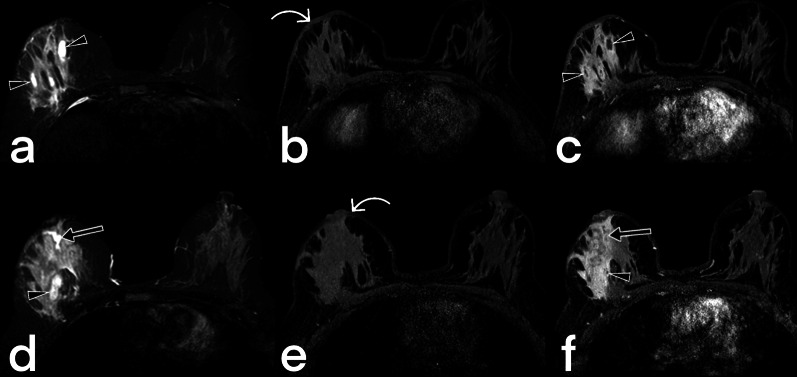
MR images of a 41-year-old female. Axial T2 weighted (a, d), pre-contrast axial T1 weighted (b, e), and post-contrast axial T1 weighted (c, f) images reveal diffuse increased signal intensity, cystic lesions (arrowheads), ductal ectasia (arrow), and thickened skin (curved arrow) in the right breast. Diffuse-type breast TB
